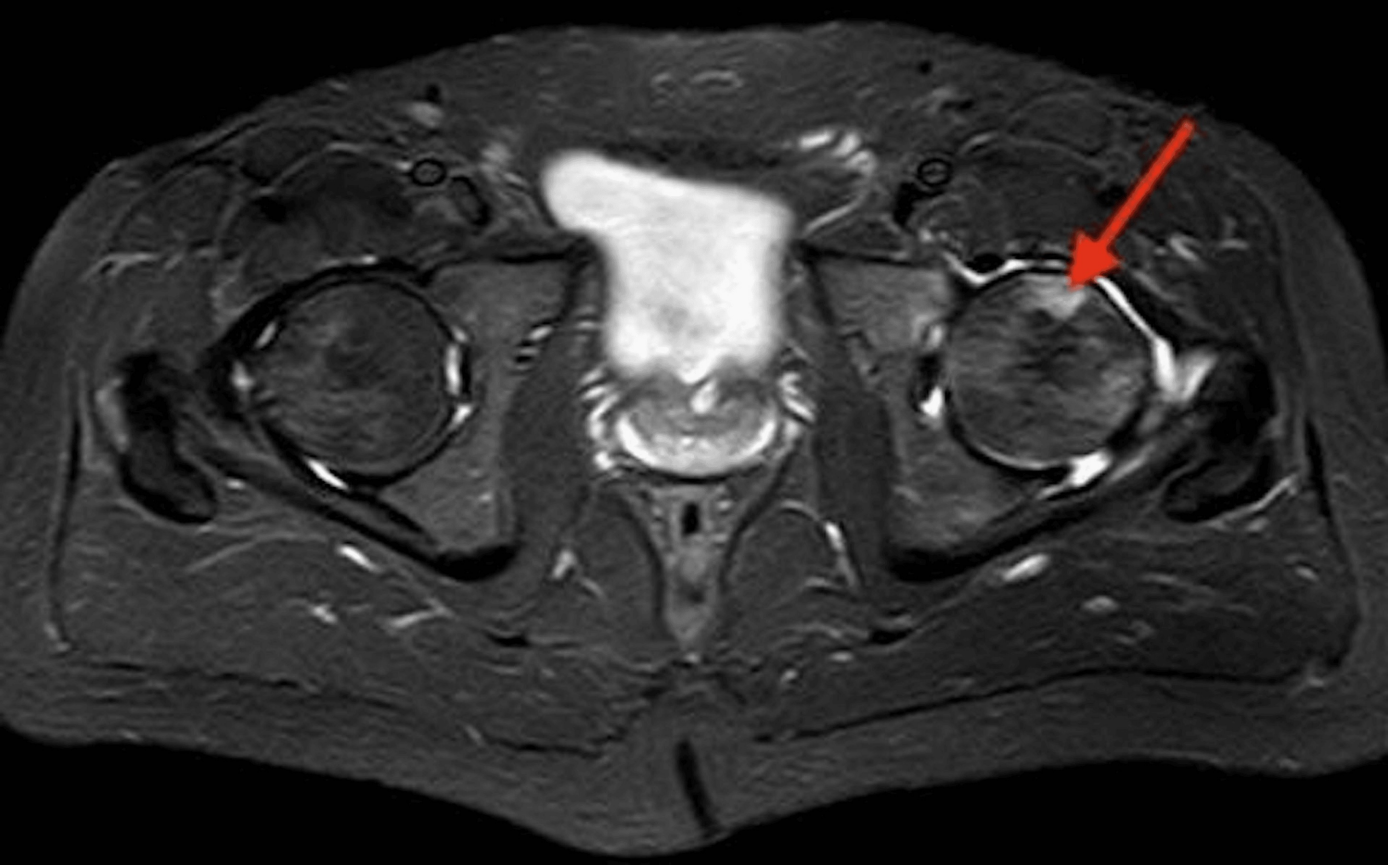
MRI of the pelvis showing normal right hip joint with bone marrow edema in the left acetabulum, femoral head, and neck Red arrow: left hip joint effusion and edema in the femoral head, neck, and acetabulum MRI: magnetic resonance imaging
